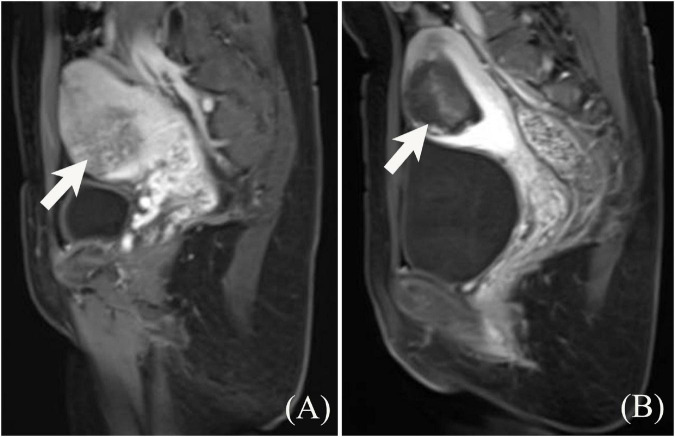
Contrast enhanced MRI of A 23-year-old woman with placenta increta before and after HIFU treatment. (A) The depth of placenta invasion was 3.7 cm, close to the uterine serous surface, and uniform enhancement of the implanted placenta with perfusion slightly below the myometrium. (B) After HIFU treatment, contrast enhanced MRI shows no perfusion of the placental tissue.
